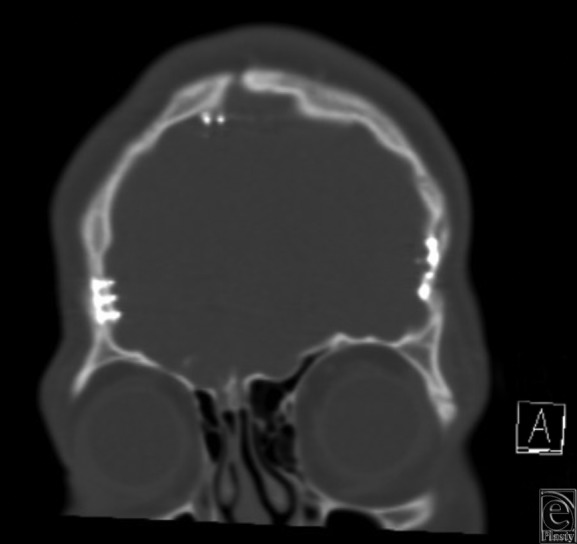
Coronal computed tomographic scan of intracranial extension of hardware.
